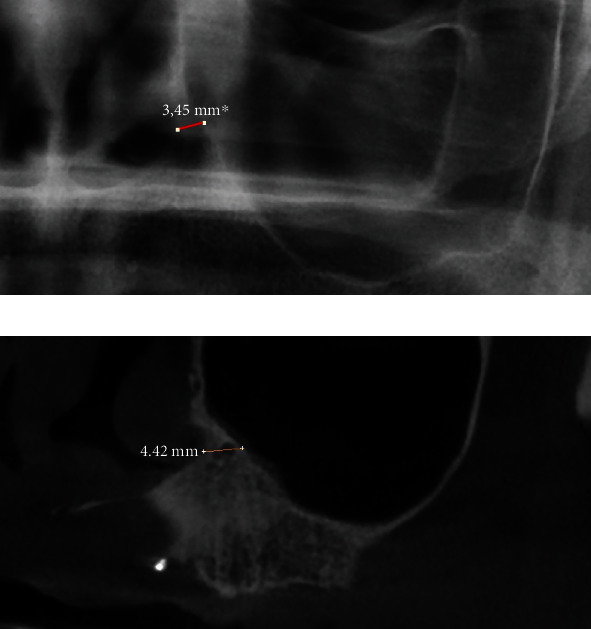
Measurement of the shortest vertical distance between the left lateral nasal wall and left maxillary sinus on a panoramic image (a) and a cone beam computed tomography image (b).
